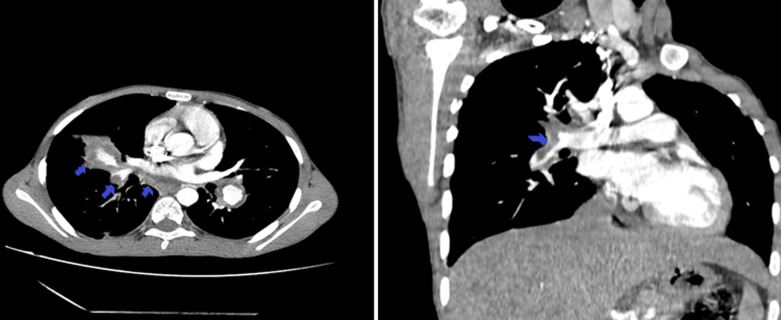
transversal and coronal CT angiography of chest showing pulmonary embolism and right ventricular thrombus
